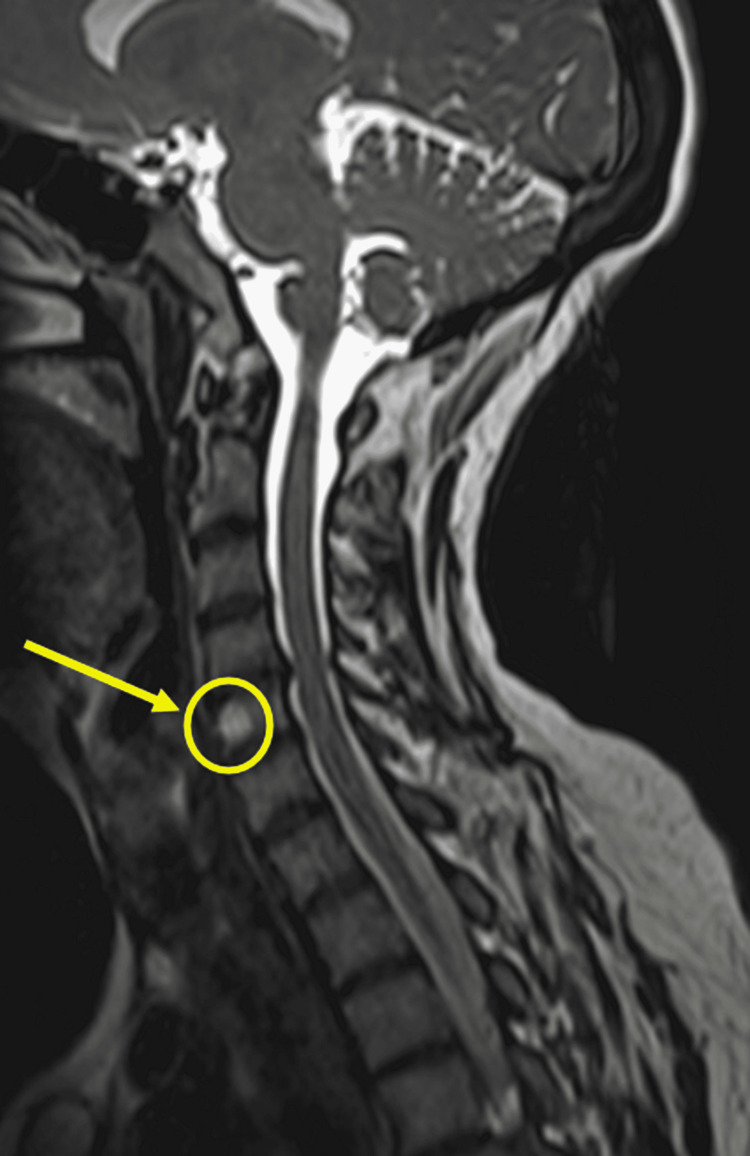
Full-body MRI showing vertebral hemangioma at level of C5 C5, L3, and L5 vertebral body interosseous hemangiomas. L3 and L5 hemangiomas are not shown in this image.
